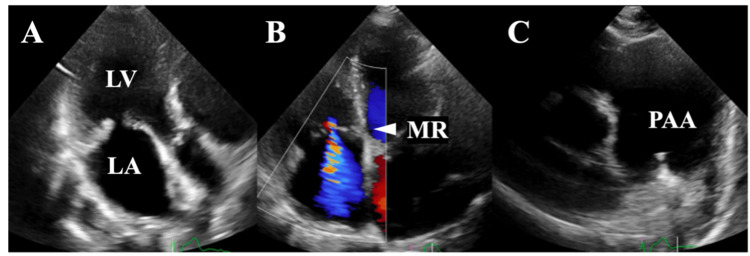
Preoperative transthoracic echocardiography Echocardiography showed moderate to severe MR with tethering of the posterior leaflet (A, B). A PAA was also observed (C). LA: left atrium; LV: left ventricle; MR: mitral regurgitation; PAA: pulmonary artery aneurysm
